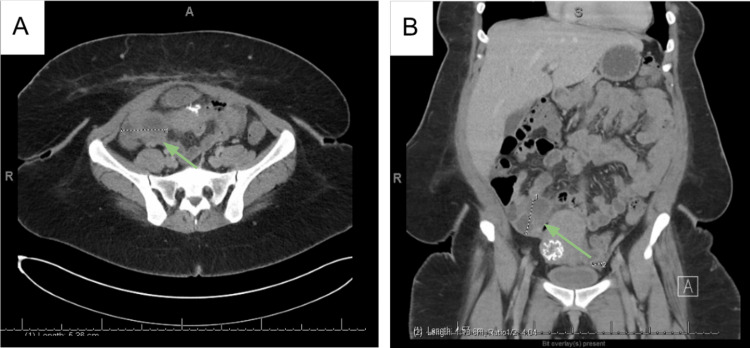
Follow-up CT scan 19 days after discharge from the hospital. CT of the abdomen and pelvis with contrast showed a multiloculated fluid collection measuring 5.3 x 4.5 cm in size [axial (A) and coronal (B) planes].
